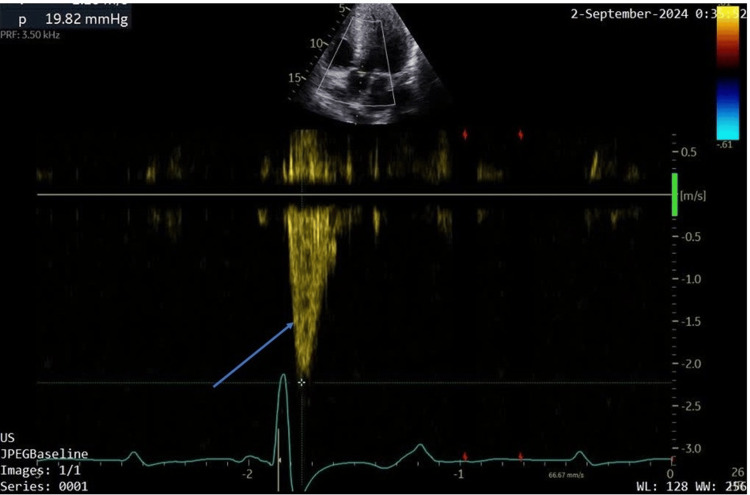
Continuous wave Doppler showing no significant gradient across the left ventricular outflow tract (blue arrow).
